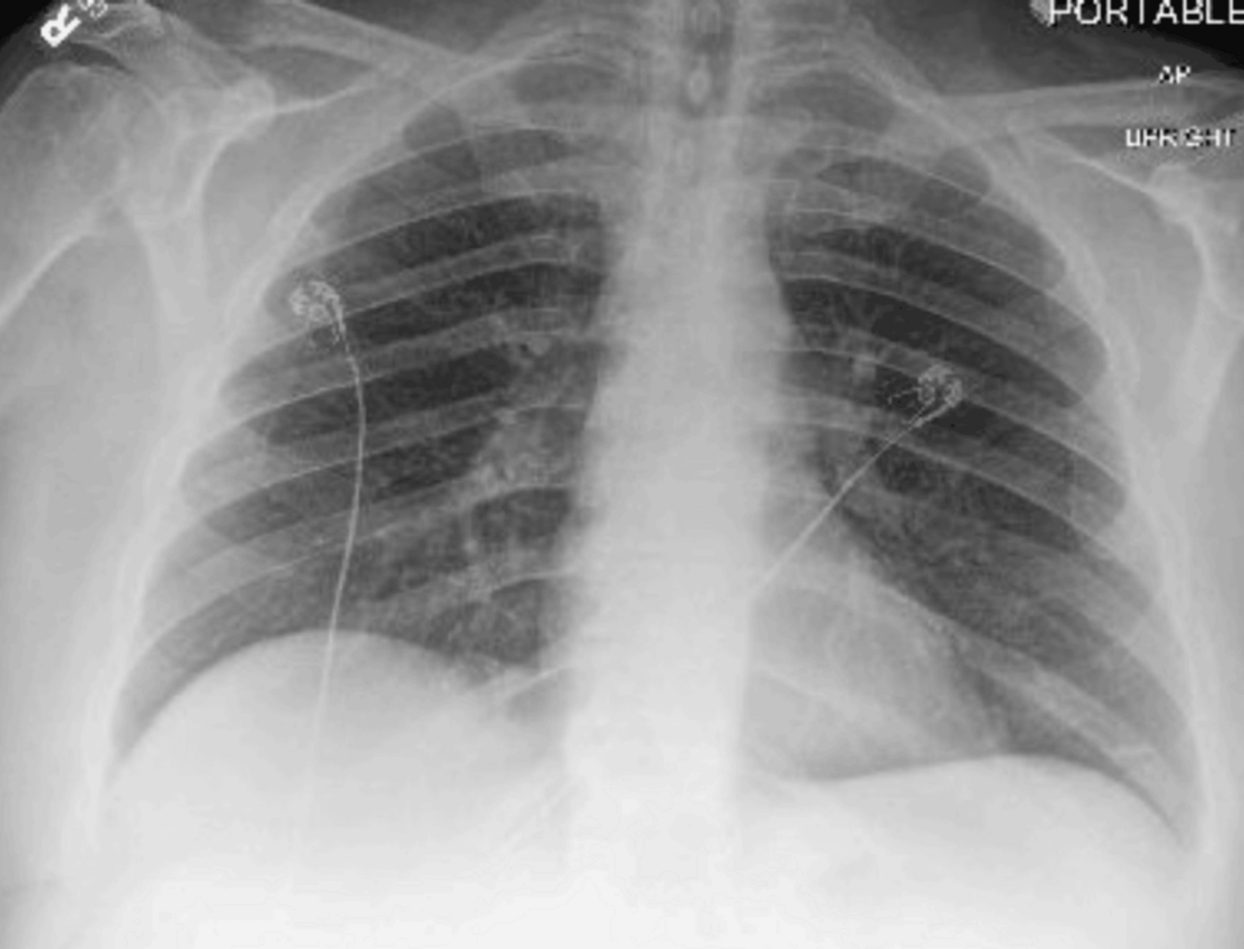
Chest X-ray at admission with no active pulmonary disease
